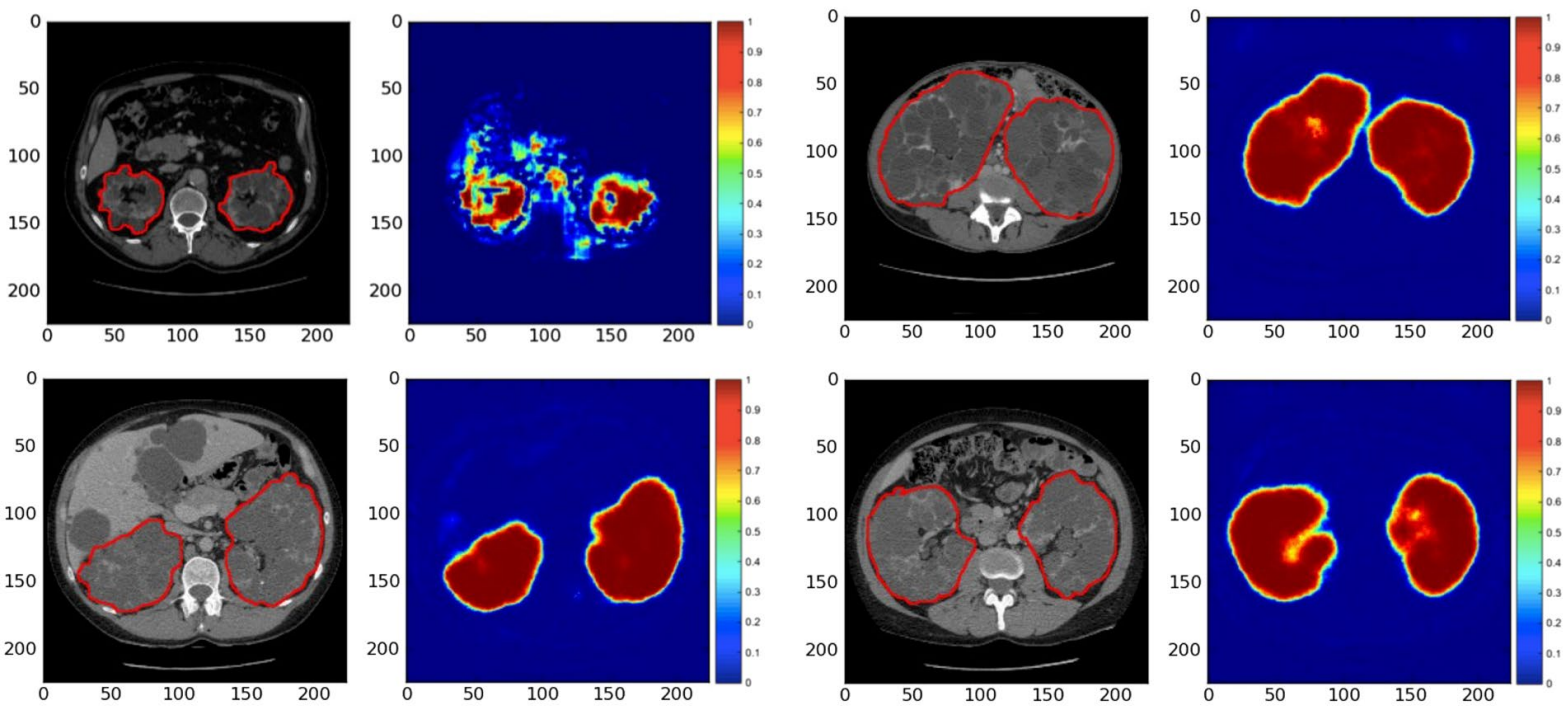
CNN predictions of ADPKD Kidneys. Four segmentations (red contour) of ADPKD kidneys from CT acquisitions of different patients are shown. The corresponding CNN-generated probability maps are shown in pseudo colors.
